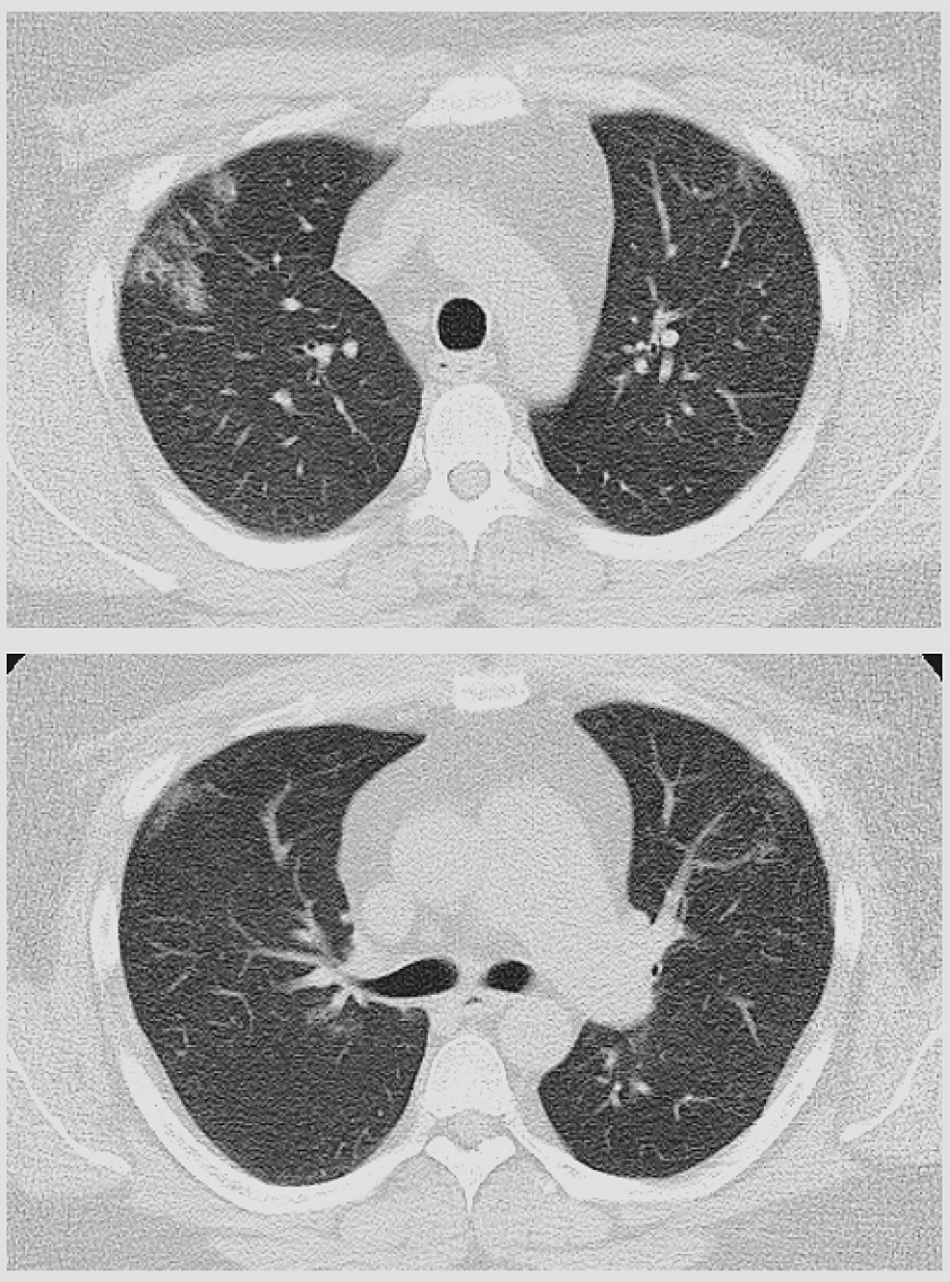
CT in January 2020 showed ground-glass opacities and infiltrative shadows in the subpleural area of the right upper lobe and ground-glass shadows in the left upper lobe. CT, computed tomography
